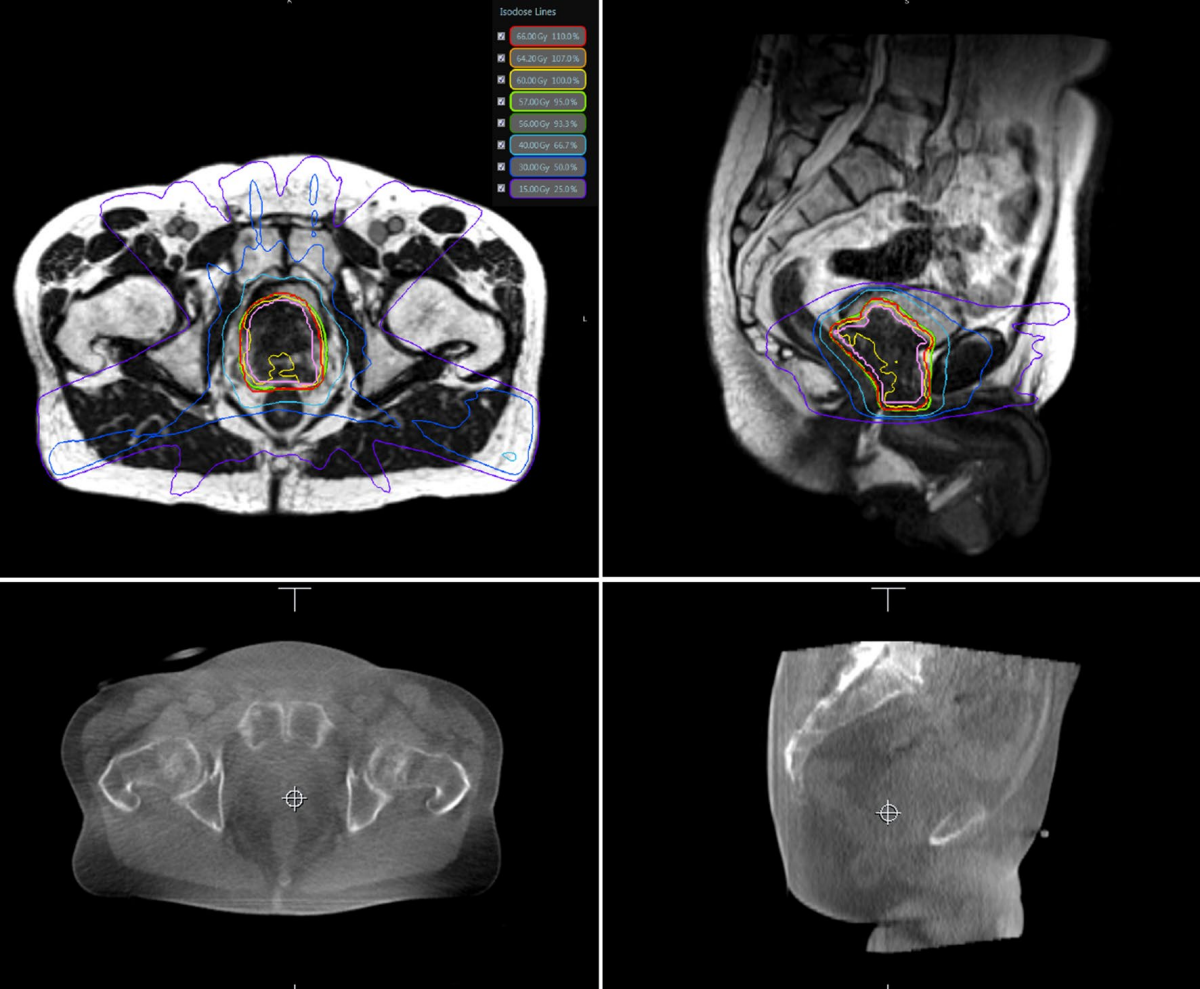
Exemplar MR-Linac based and Cone Beam CT based imaging of prostate cancer
